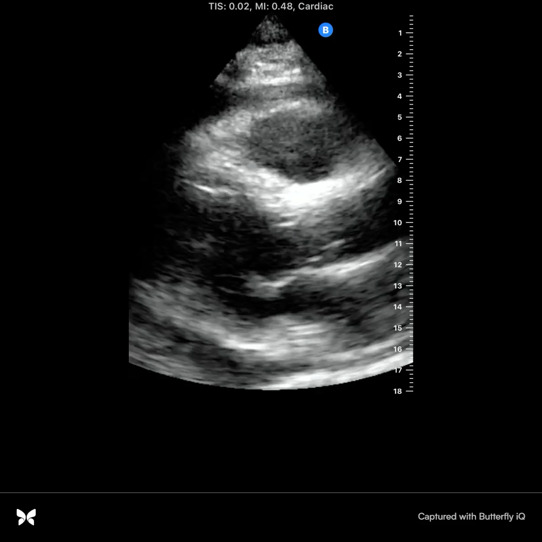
Parasternal long axis view by point of care cardiac ultrasonography revealing moderate to large pericardial effusion.
